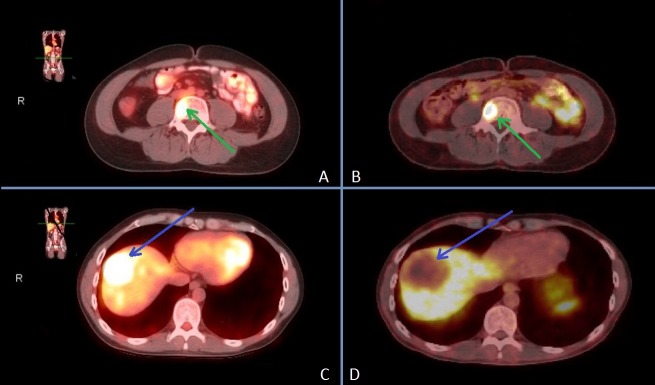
18F-FDG PET/CT and gallium-68 DOTATATE scans showing matched target lesions in liver and bone metastases. It is important to note that the lack of DOTATATE avidity in a circumscribed region of the liver is due to SBRT radiation-induced tumor necrosis A) 18F-FDG PET/CT vertebral body lesion (green arrow) B) 68Ga-DOTATOC SSTR PET/CT vertebral body lesion (green arrow) C) 18F-FDG PET/CT liver lesion prior to SBRT treatment (blue arrow) D) 68Ga-DOTATOC SSTR PET/CT liver lesion after SBRT treatment (blue arrow) 18F-FDG PET/CT: [18F] fluorodeoxyglucose  positron emission tomography/computed tomography SBRT: Stereotactic body radiation therapy SSTR: somatostatin receptor
